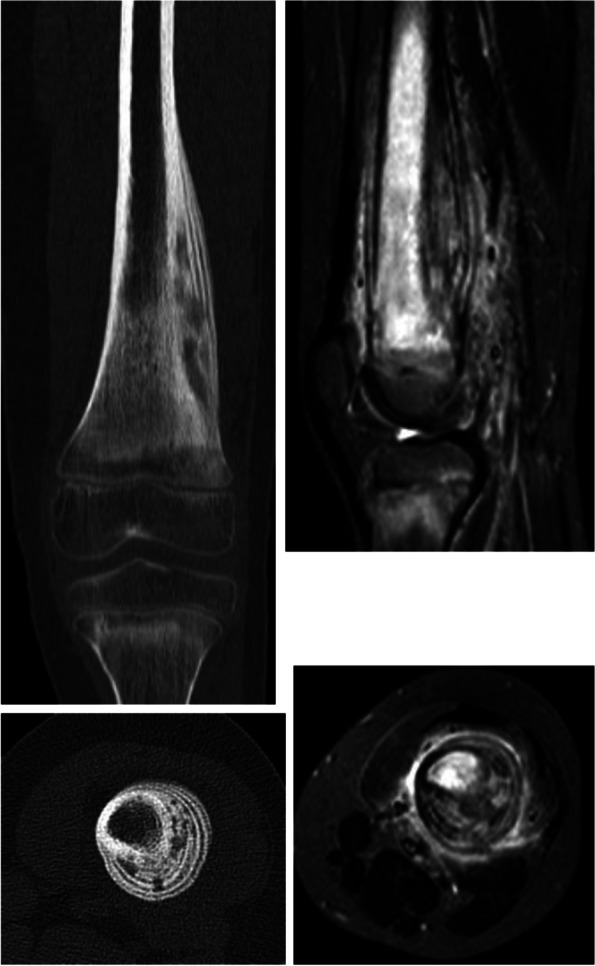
Distal femoral CNO lesion: CT and MRI imaging Left: CT image shows osteolytic region with periosteal reaction. Right: MRI image (STIR) demonstrates bone marrow edema and surrounding soft tissue edema and layers of periosteal reaction
